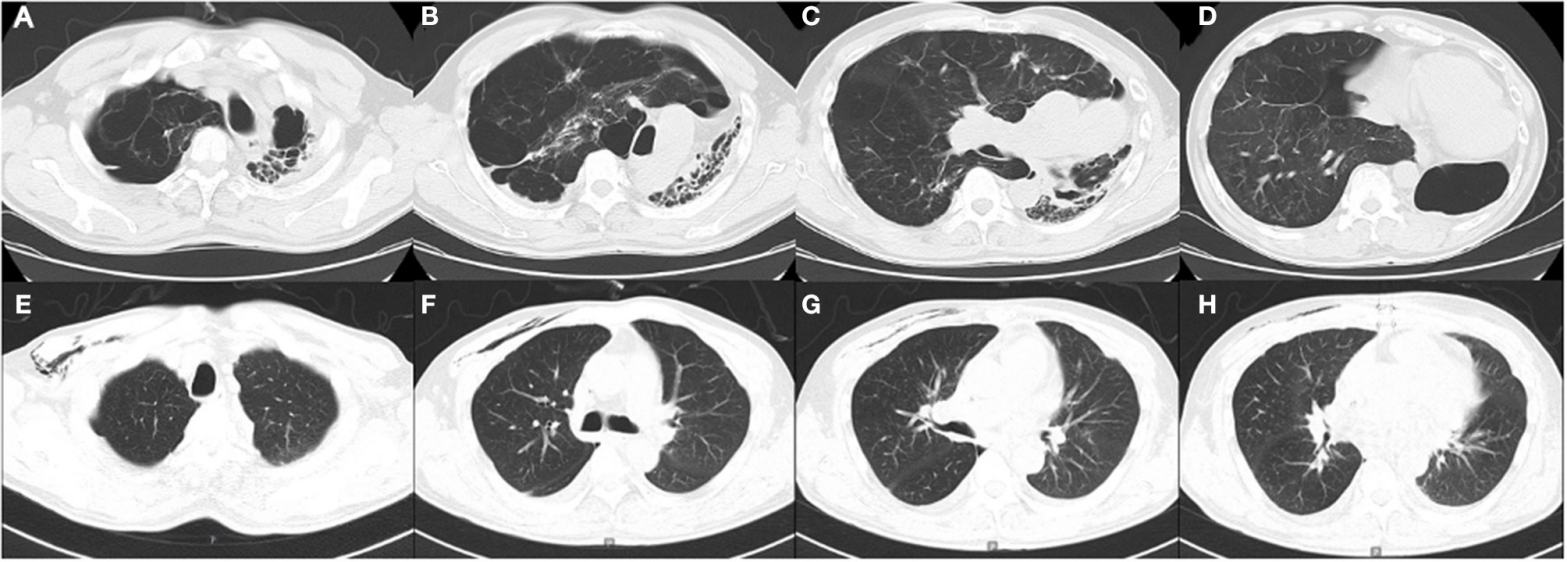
(A–D). Preoperative computed tomography (CT) images of patient 1. (E–H). Postoperative CT images of patient 1 one month after the surgery.
